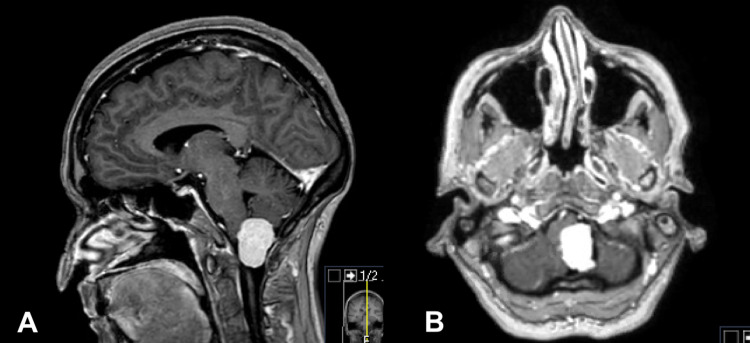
Post-contrast sagittal (A) and axial (B) MRI scans. The scans demonstrating a 23×16×22 mm intradural, extramedullary lesion with medullary and upper cervical cord compression.
